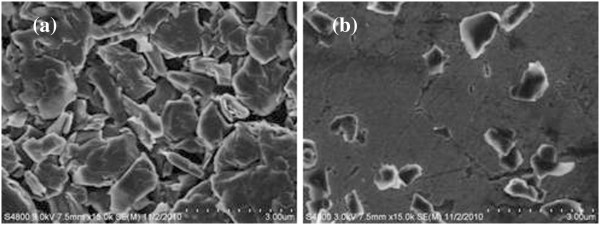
Effects of ultrasonic pretreatment on graphite particles. (a) Before ultrasonic pretreatment and (b) after ultrasonic pretreatment.
